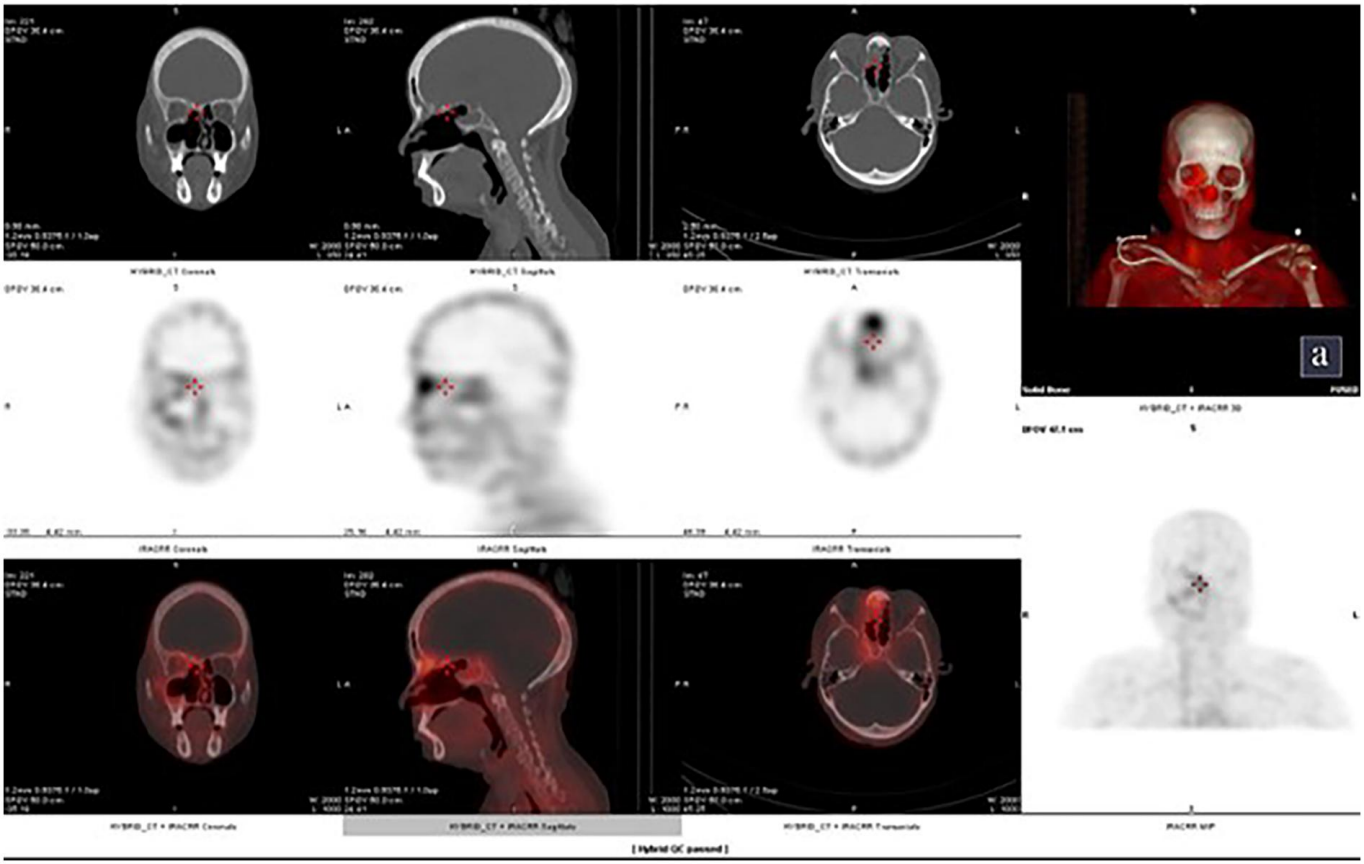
Case 1: additional SPECT/CT images showing involvement of right frontal bone, nasal septum, lesser wing of sphenoid on the right side, and body of sphenoid.
